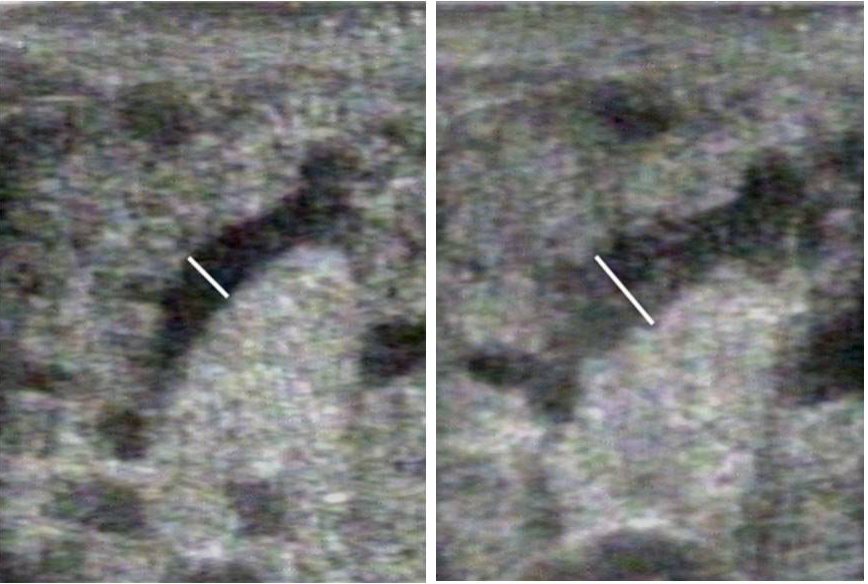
Milk duct diameter increase, as measured by ultrasound. These images depict a milk duct increasing in duct diameter at the time of milk ejection.
